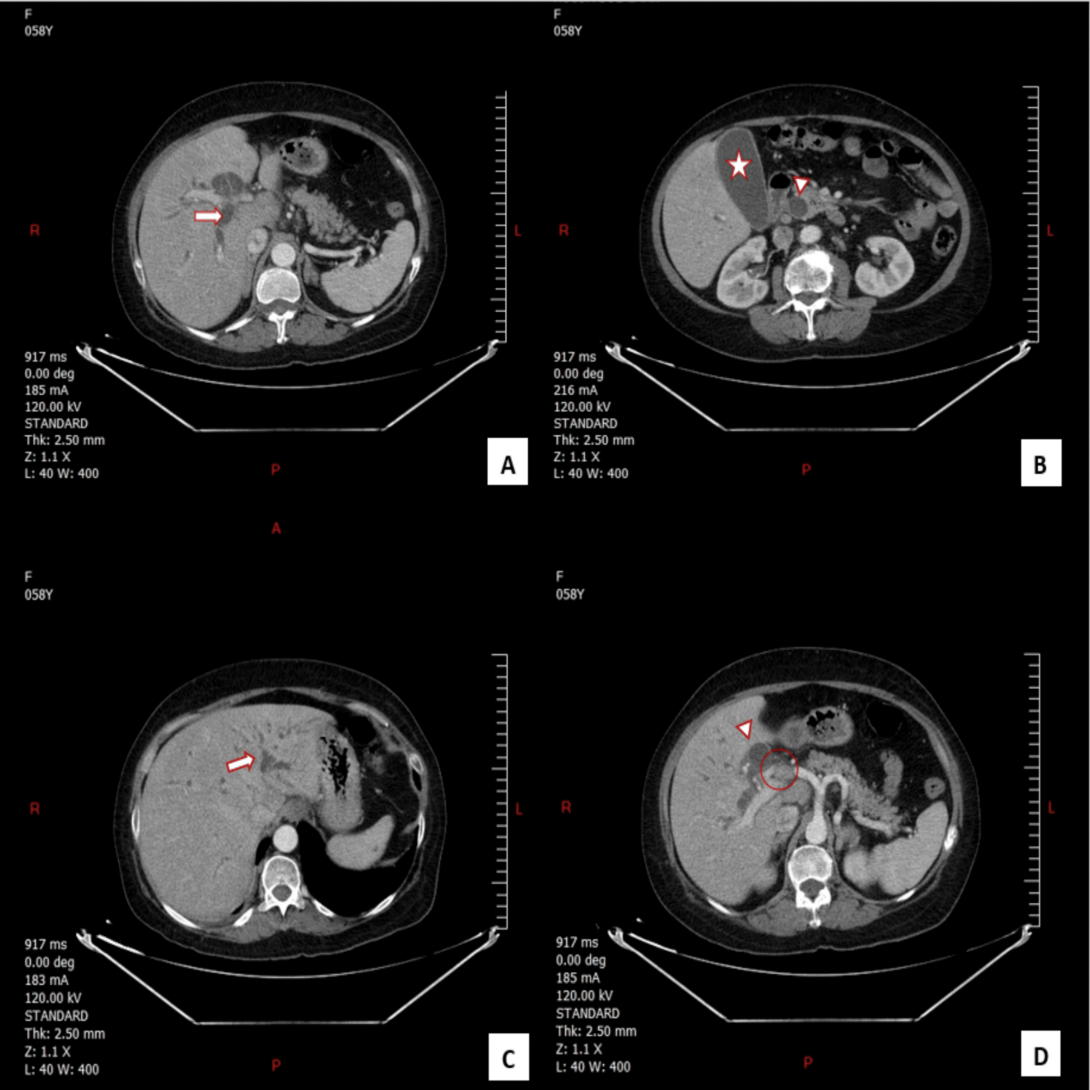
Contrast-enhanced CT scan of the abdomen revealing a mass consistent with obstructing cholangiocarcinoma Figures 1A, 1B, and 1C: CT imaging demonstrates significant dilation of the intra-hepatic (arrowhead) and extra-hepatic(arrow) bile ducts, with accompanying distension of the gallbladder (star), consistent with biliary obstruction secondary to cholangiocarcinoma. Figure 1D: An enlarged lymph node at the hepatic hilum is noted (circle), along with gallbladder distension, raising suspicion for regional metastasis and biliary obstruction in the context of cholangiocarcinoma.
